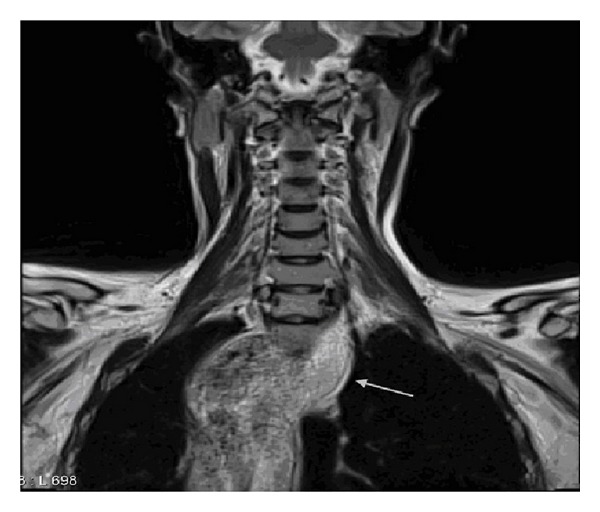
MRI scan of the neck region showing the extent of the esophageal dilatation (arrow) (coronal view).
